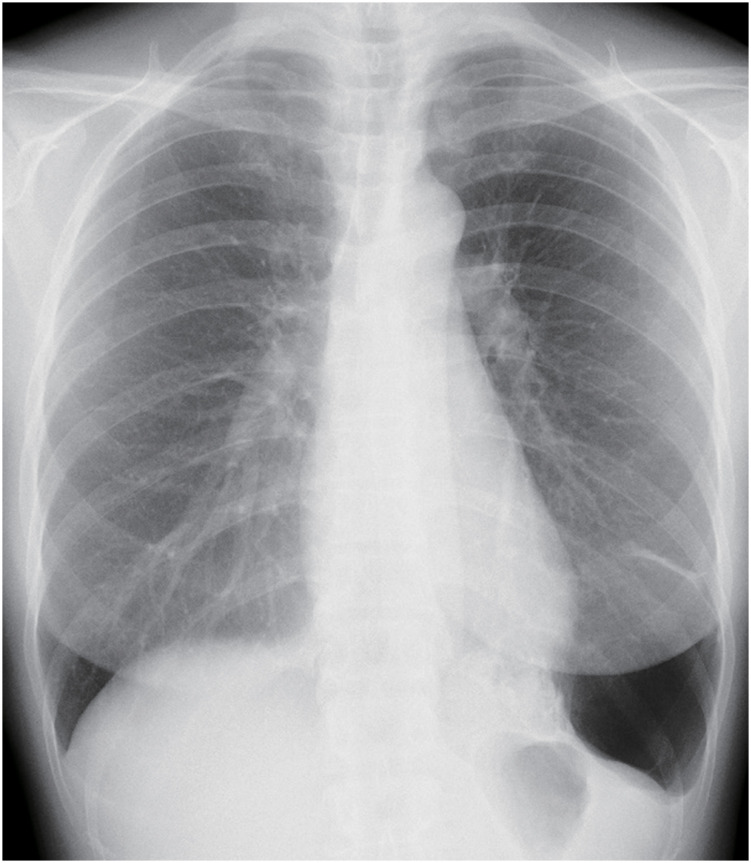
X-ray photograph taken during the initial health examination. Abnormalities with increased permeability were observed in the left lower lung field.
